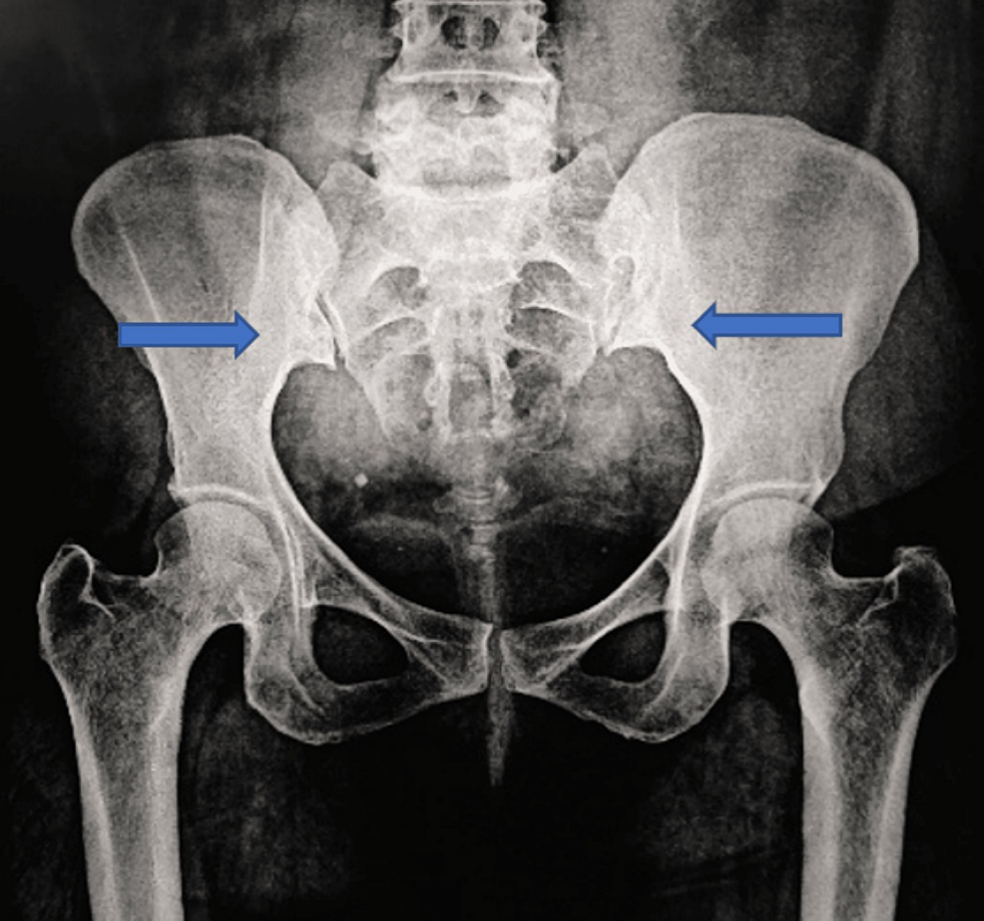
Antero-posterior radiograph of pelvis showing sclerosis at iliac border of sacroiliac joints.
